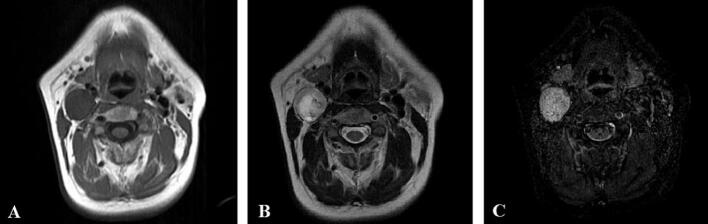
MRI scan in axial plane indicating neoplasm of carotid sheath of the right side of neck. A- T1, B- T2, C- T2 STIR sequences.
